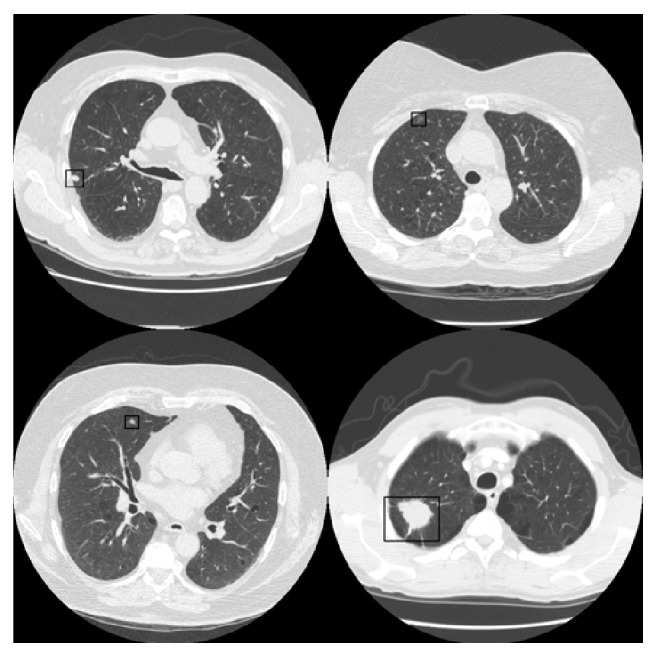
Different helical thoracic CT scans used in the tests with the nodule marked by a black rectangle.
